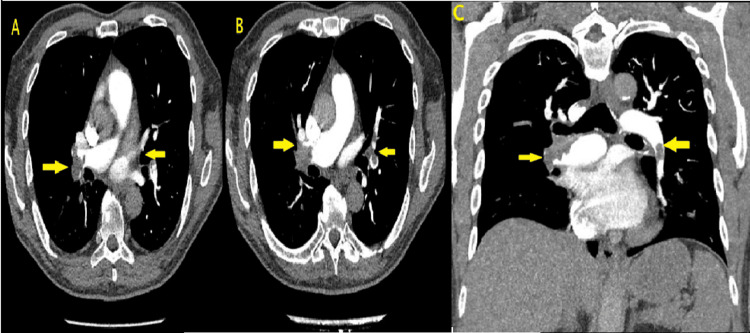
CTPA showing bilateral pulmonary emboli Axial (A,B) and coronal (C) views of the pulmonary arteries showing bilateral pulmonary emboli in the right and left subsegmental pulmonary arteries CTPA: computed tomography pulmonary angiogram
